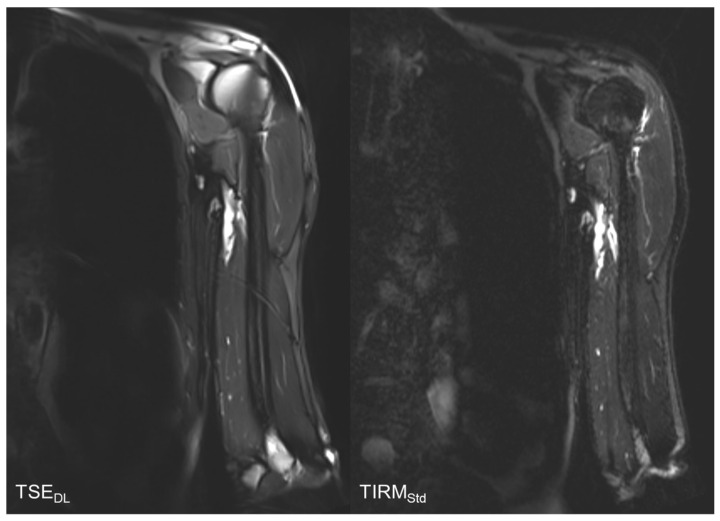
Comparison of T2-weighted TSE_DL_ and T2 TIRM_Std_. The T2 TIRM_Std_ shows a better image quality, contrast, and sharpness.
